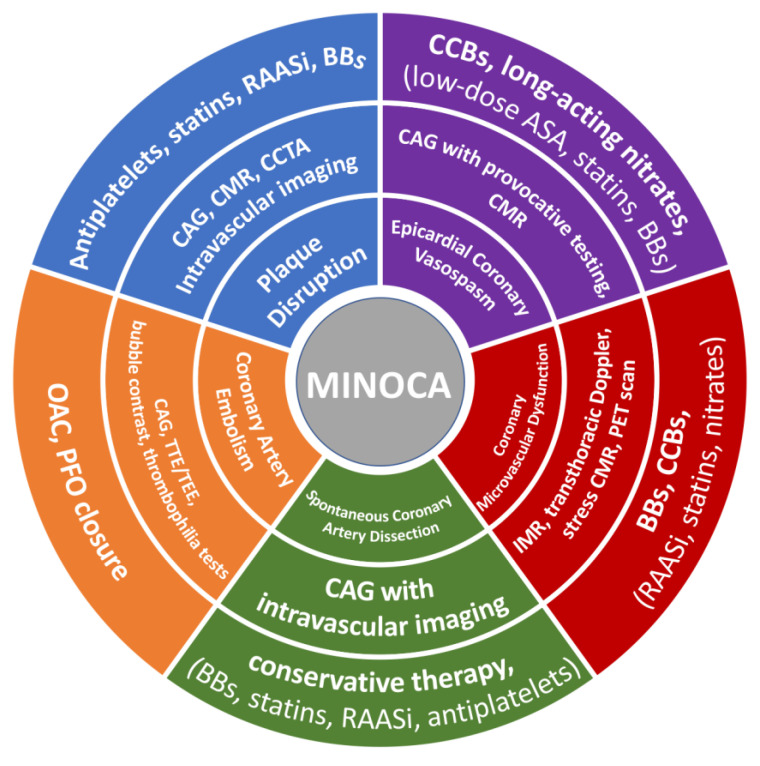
Diagnostic tools and therapeutic strategy based on the etiology of MINOCA. CAG—coronary angiogram; CMR—cardiac magnetic resonance; CCTA—coronary computed tomography angiography; ASA—acetylsalicylic acid; BBs—beta-blockers; RAASi—renin–angiotensin adrenergic antagonists; CCBs—calcium channel blockers; OAC—oral anticoagulation; PFO—patent foramen ovale; TTE—transthoracic echocardiography; TEE—transesophageal echocardiography; PET—positron emission tomography; IMR—index of microvascular resistance.
